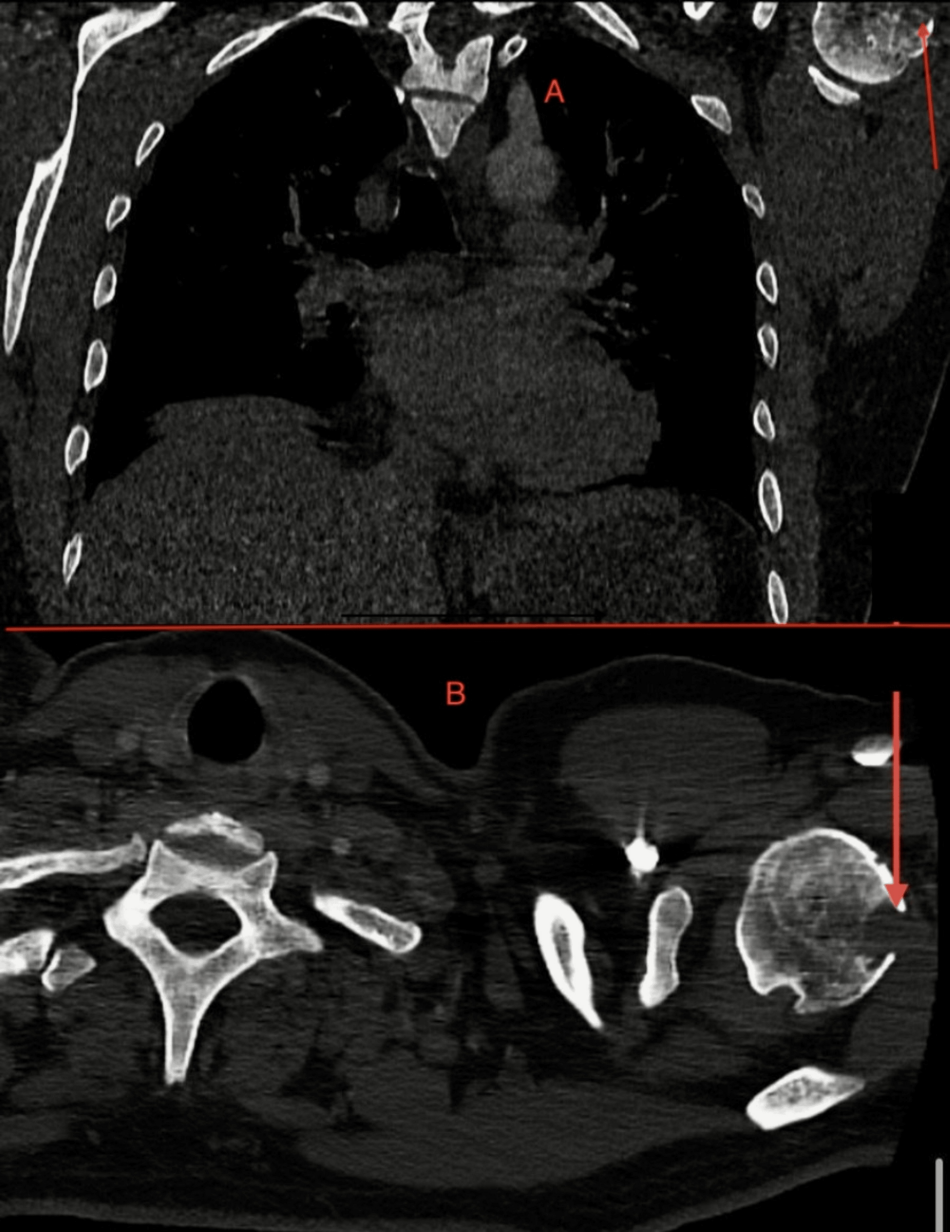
CT of the chest and shoulder demonstrating a 15×11 mm lytic lesion in the left humeral head with cortical rupture A: coronal CT scan showing a bone lesion in the upper thoracic region compatible with granulomatous involvement. B: axial CT scan demonstrating a lytic mass of the right scapula corresponding to osseous sarcoidosis involvement.
